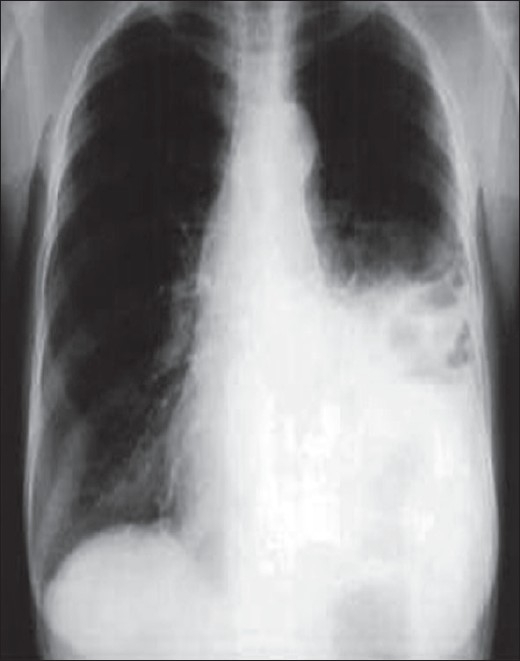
Standard AP chest X-ray depicting an elevated diaphragm with loculation of the left pleural space
